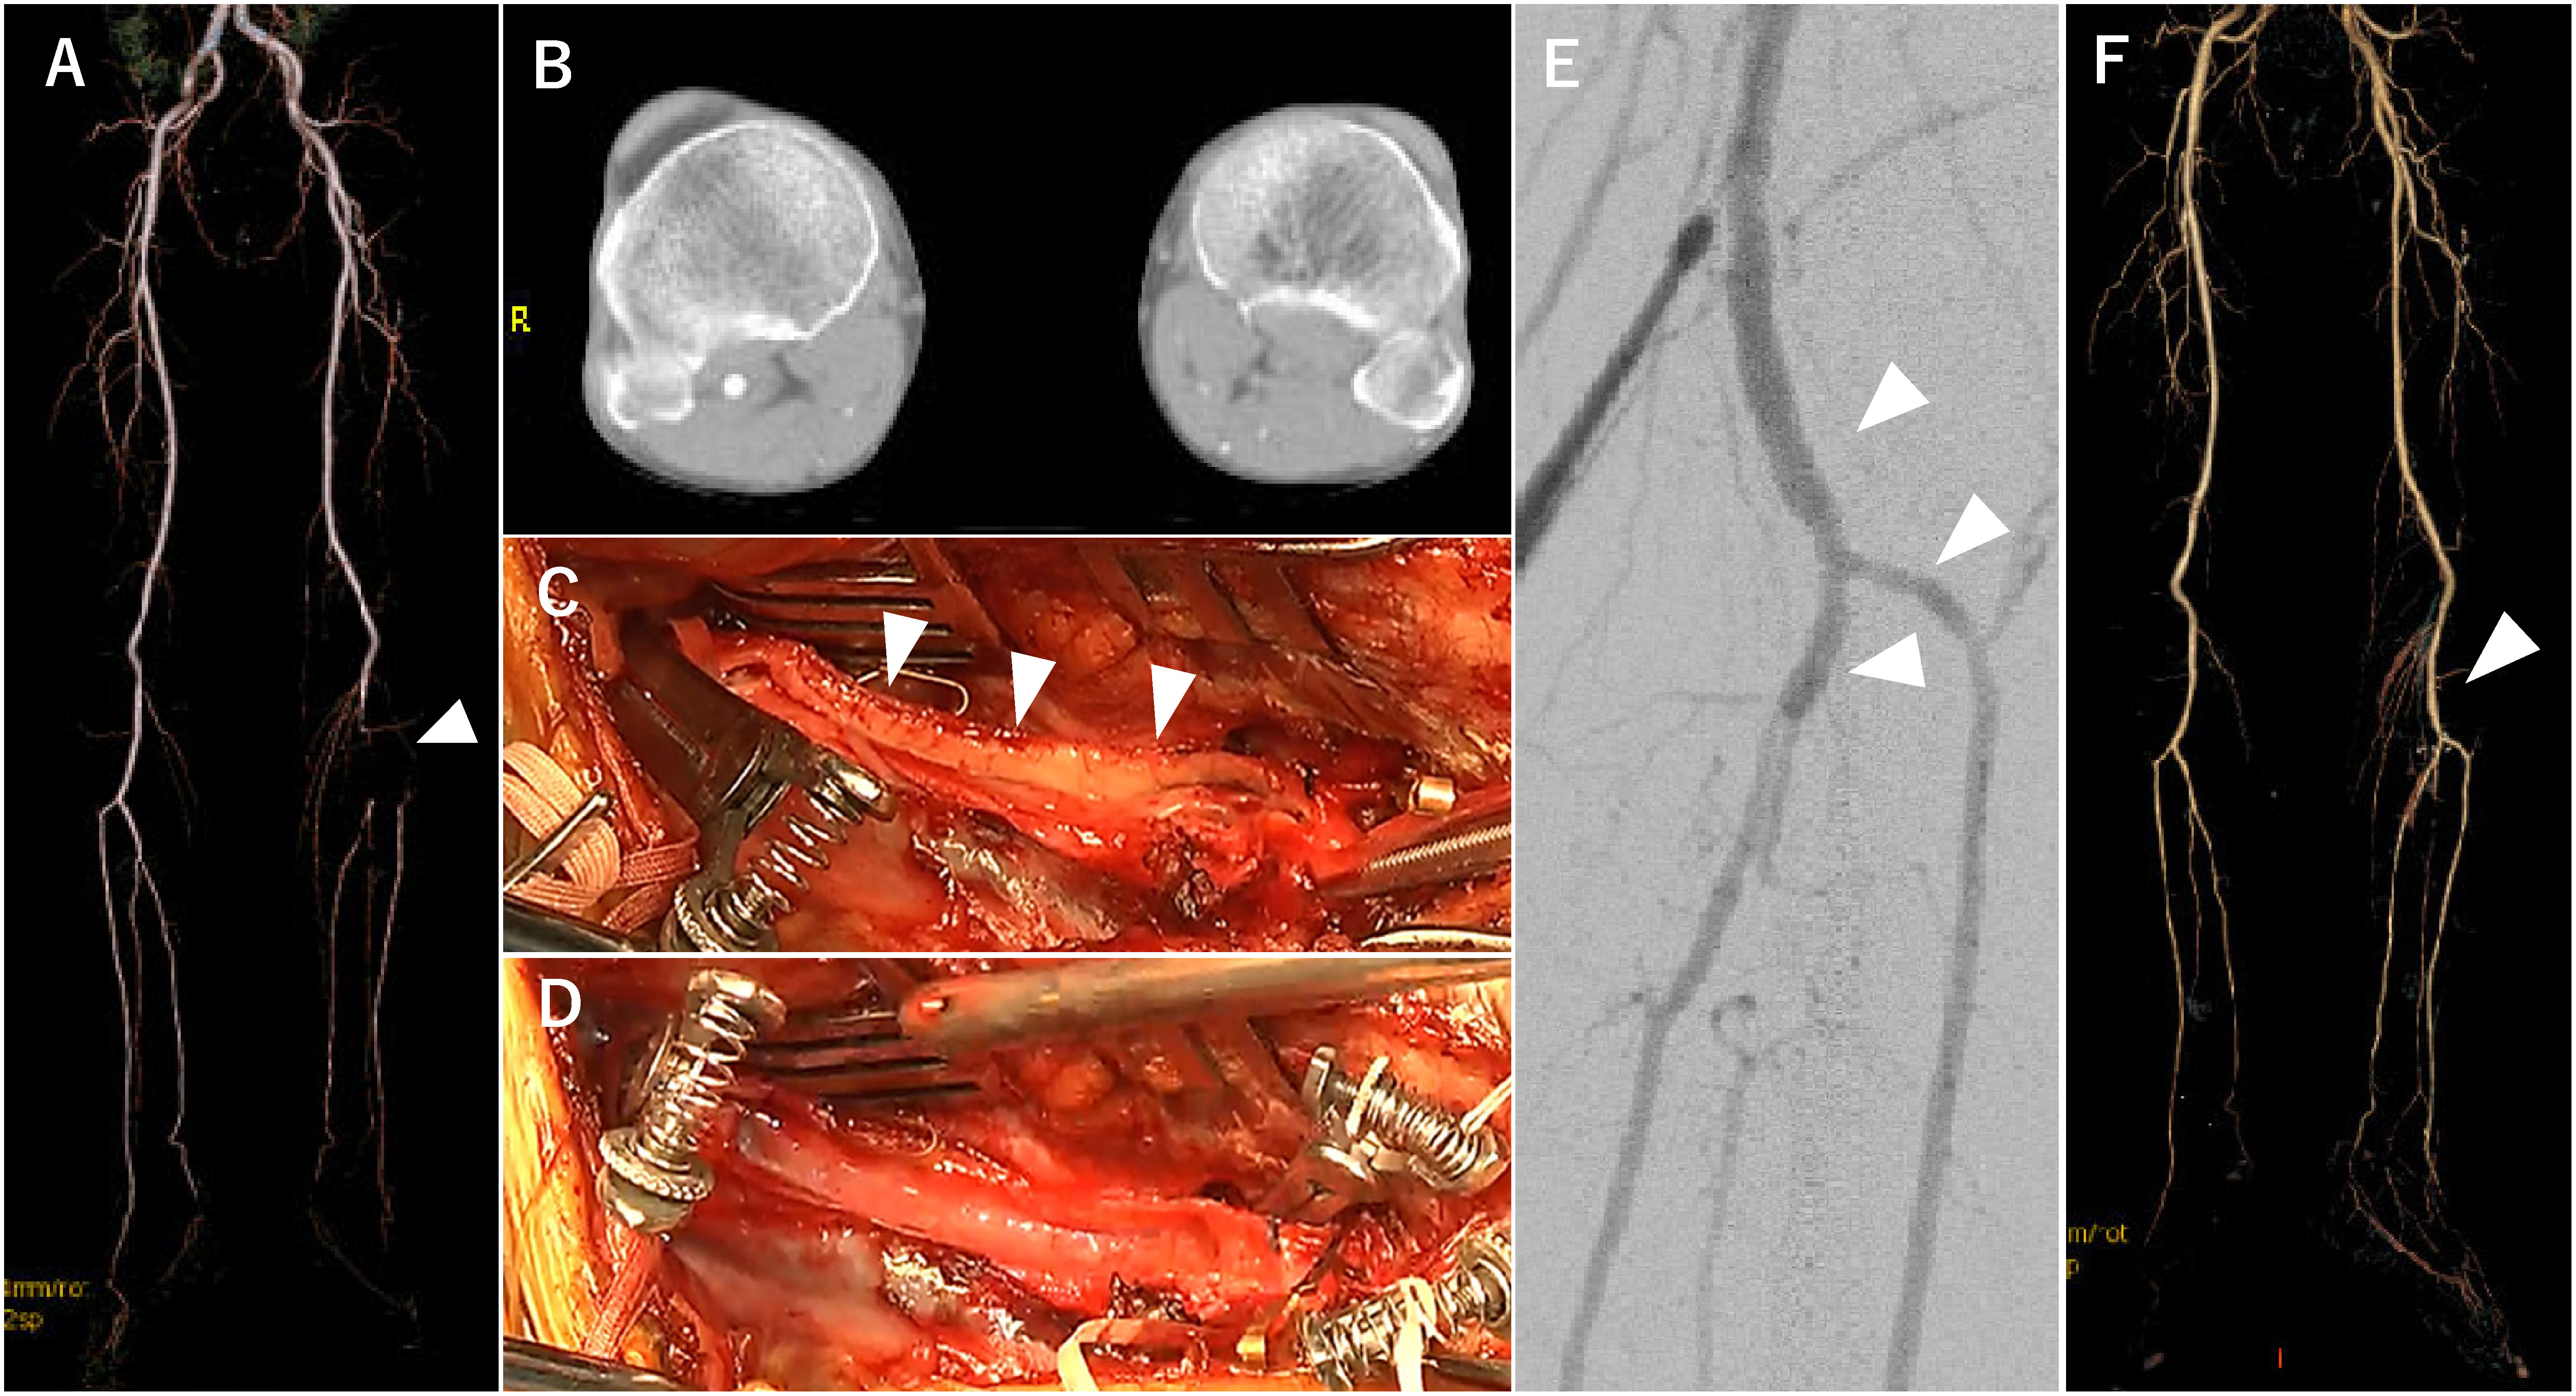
Fig. 1 Preoperative computed tomography angiography (CTA) showed occlusion of the left infragenicular arteries. The focal occlusive lesions involved the left distal popliteal artery and the trifurcation (arrowhead) (A). Axial imaging negated the possibility of popliteal artery entrapment and cystic adventitial disease of the popliteal artery (B). Intraoperative findings and postoperative CTA. Thickened walls were found in the infragenicular arteries, and soft and white thrombi were observed in the popliteal artery and tibioperoneal trunk (arrowheads) (C). Endarterectomy of 5 cm in length was carefully performed (D). The intraoperative angiography showed a well-recanalized popliteal artery, tibioperoneal trunk, and anterior tibial artery (arrowheads) (E). Postoperative CTA (1 week after the surgery) showed good revascularization of the infragenicular occlusive lesions (arrowhead) (F).
